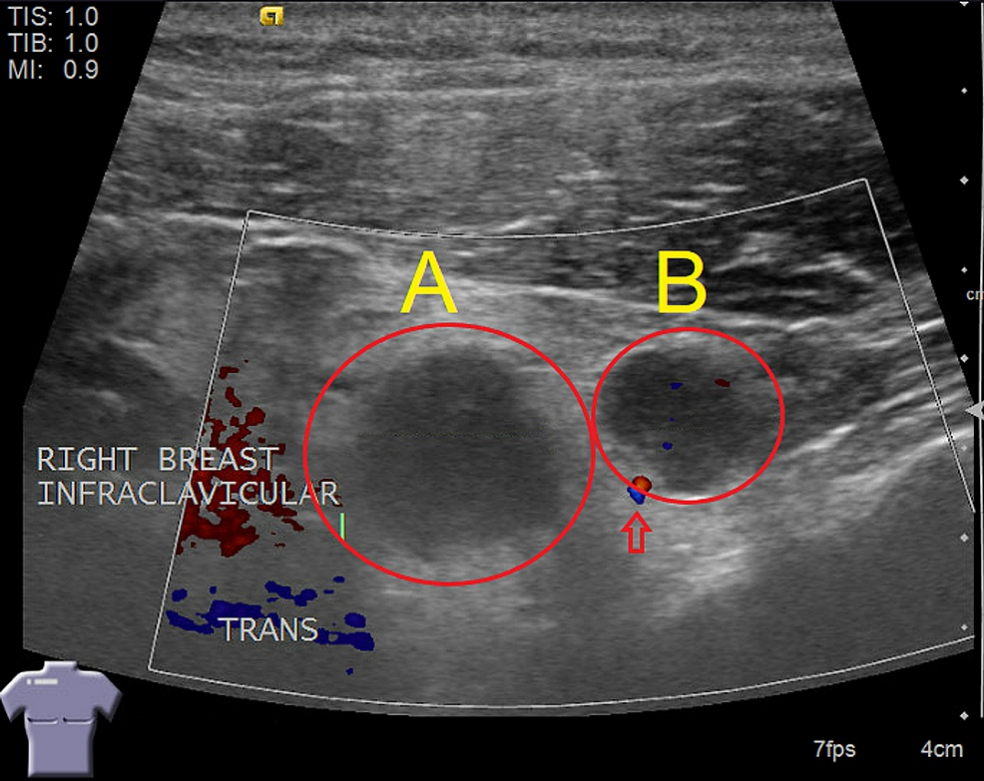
Right Infraclavicular Ultrasound Image shows two adjacent abnormal appearing infraclavicular lymph nodes (red circles) which measure 10 mm x 9 mm x 12 mm (A) and 8 mm x 10 mm x 12 mm (B) with mild associated increased vascularity (arrow).
